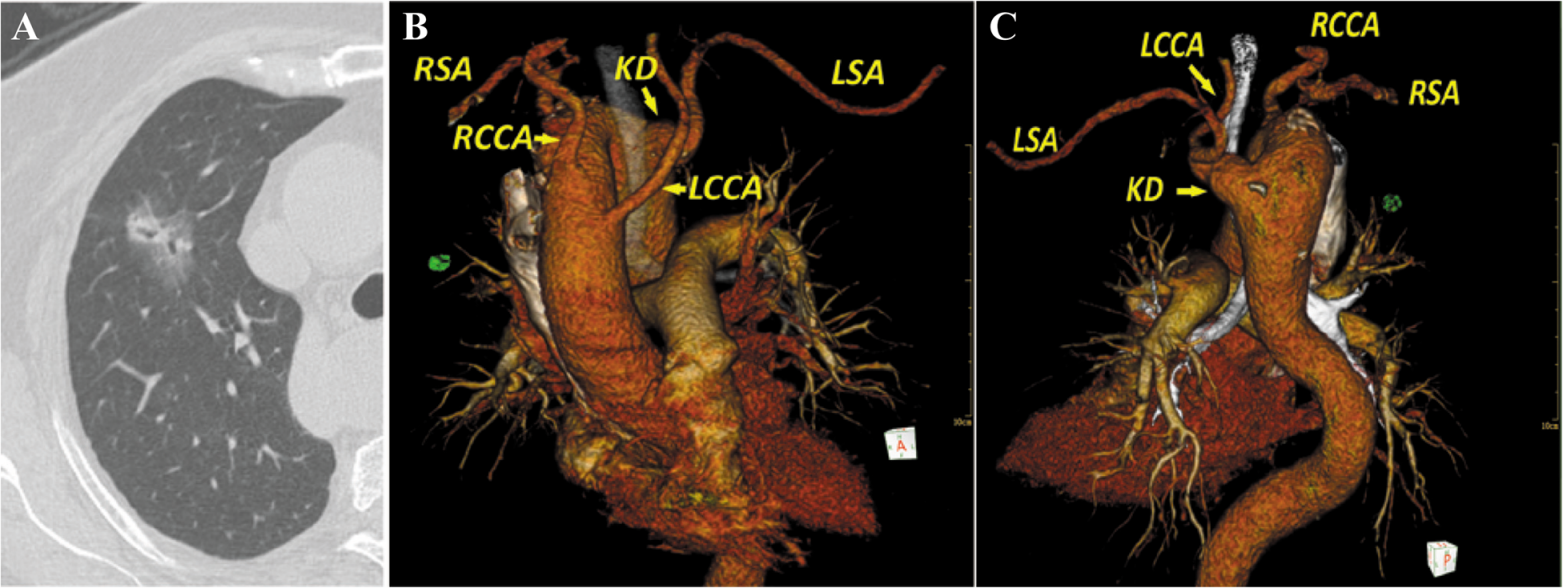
a Preoperative CT reveals a 34 mm × 20 mm partial solid nodule at the right S3. b The anterior view of the 3D CT image shows the right-sided aortic arch with the aberrant left subclavian artery. c The posterior view of the 3D CT image shows the Kommerell diverticulum of the left subclavian artery. RSA, right subclavian artery; RCCA, right common carotid artery; LSA, left subclavian artery; LCCA, left common carotid artery; KD, Kommerell diverticulum
